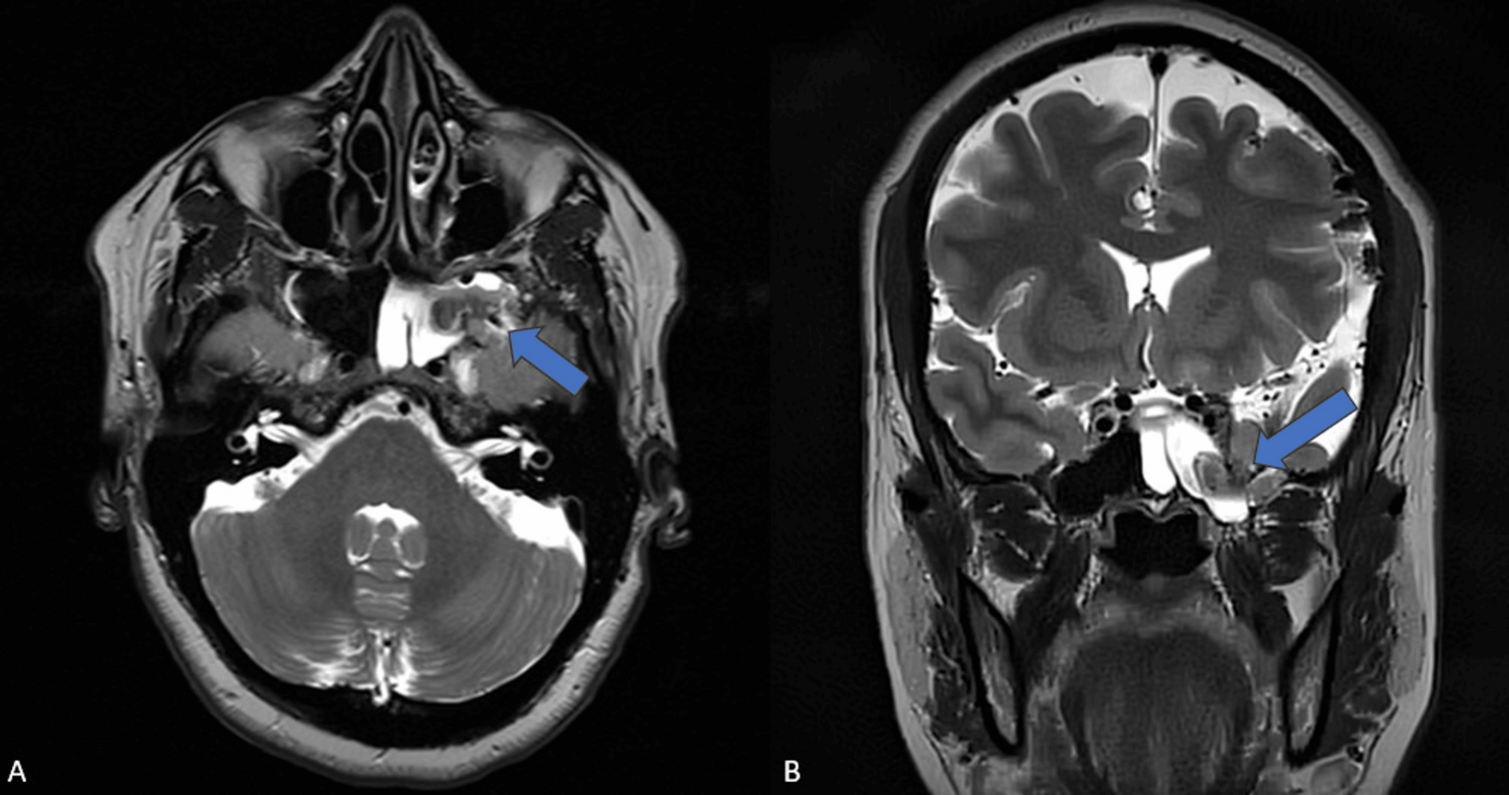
MRI brain axial and coronal T2W image at the level of the sphenoid sinus A: axial T2W MRI brain; B: coronal T2W MRI brain shows a defect (blue arrow) in the left lateral recess of the sphenoid sinus with herniation of meninges, CSF, and a portion of the left anterior temporal lobe.
